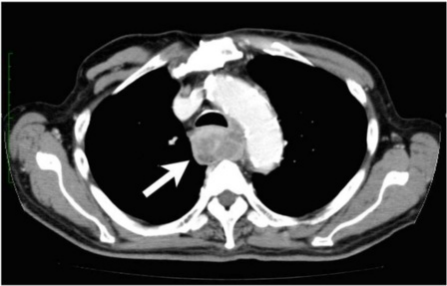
Computed tomography scan of thoracic esophageal tumor growing in the middle portion of the mediastinum at the time when the patient suffered from severe dysphagia
